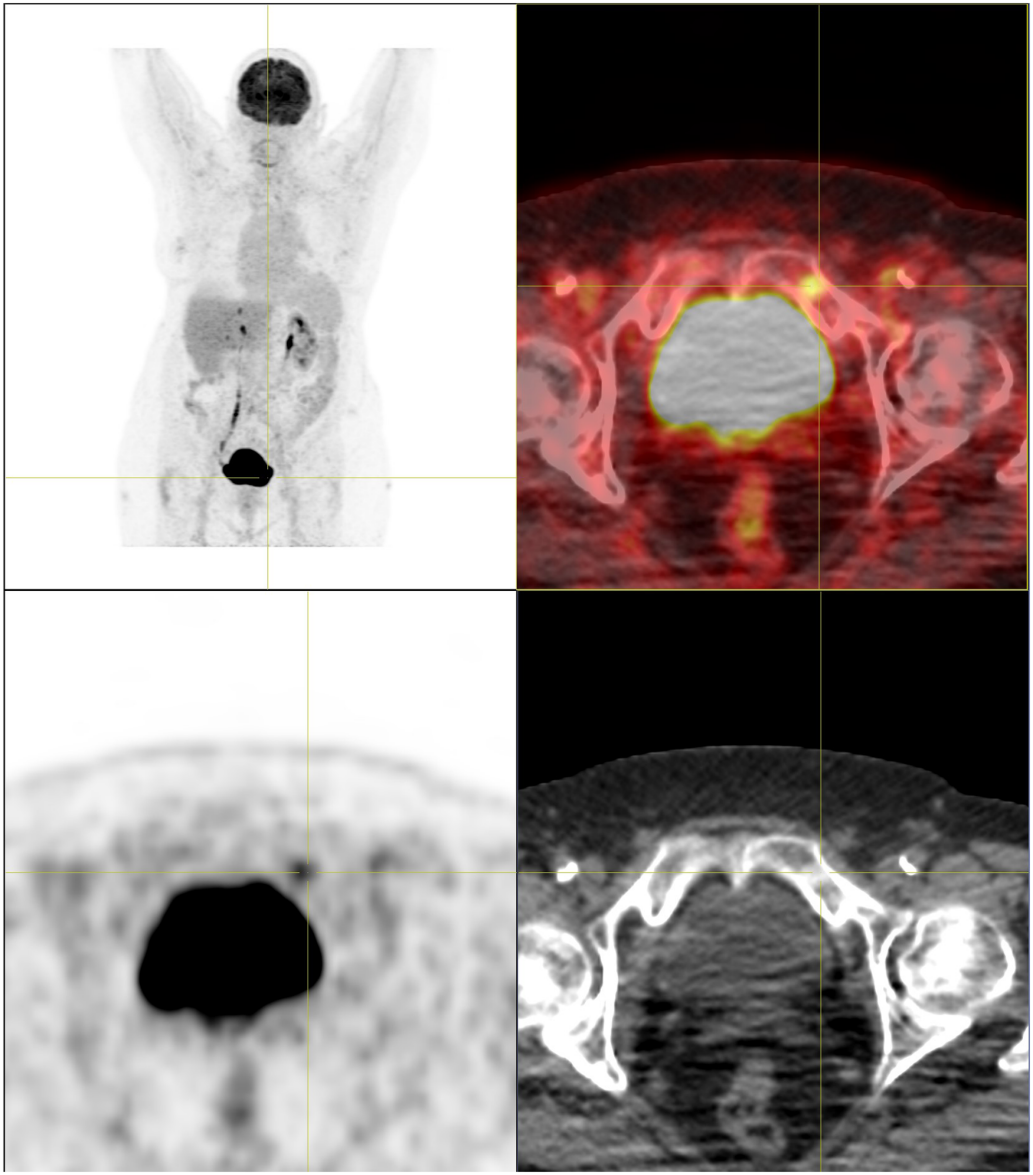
FDG-PET/CT. Roundish sclerosing lesion with low FDG-uptake in the upper left pubic branch.
